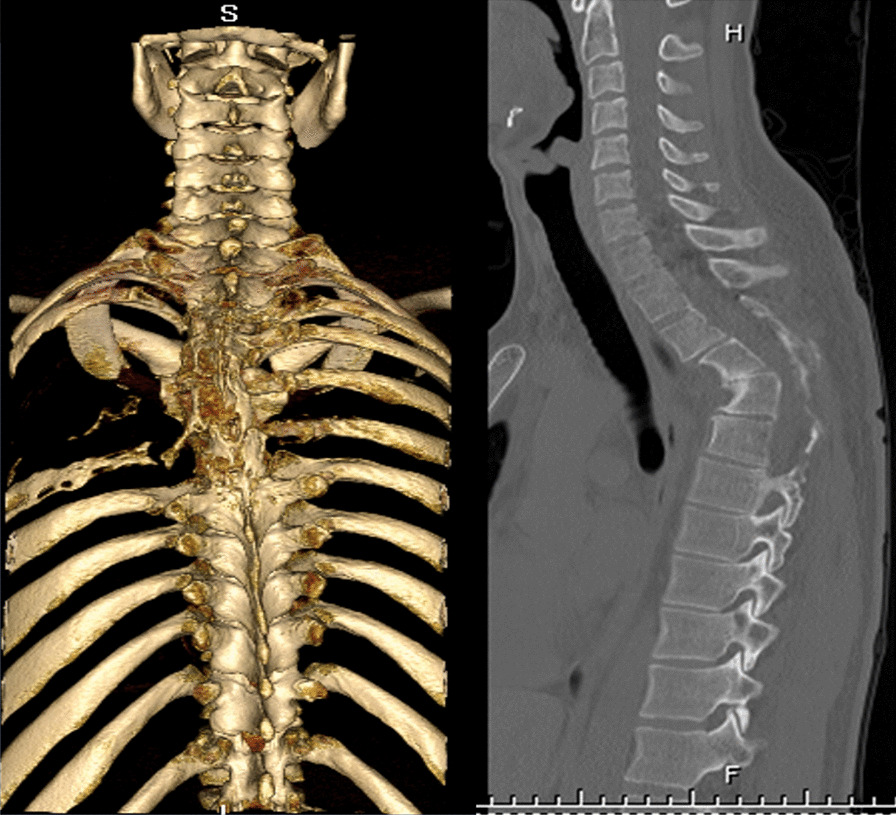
Computed tomography showing destruction of left IV–VI ribs and thoracic vertebrae III–IV together with kyphosis and spinal stenosis of thoracic spine
